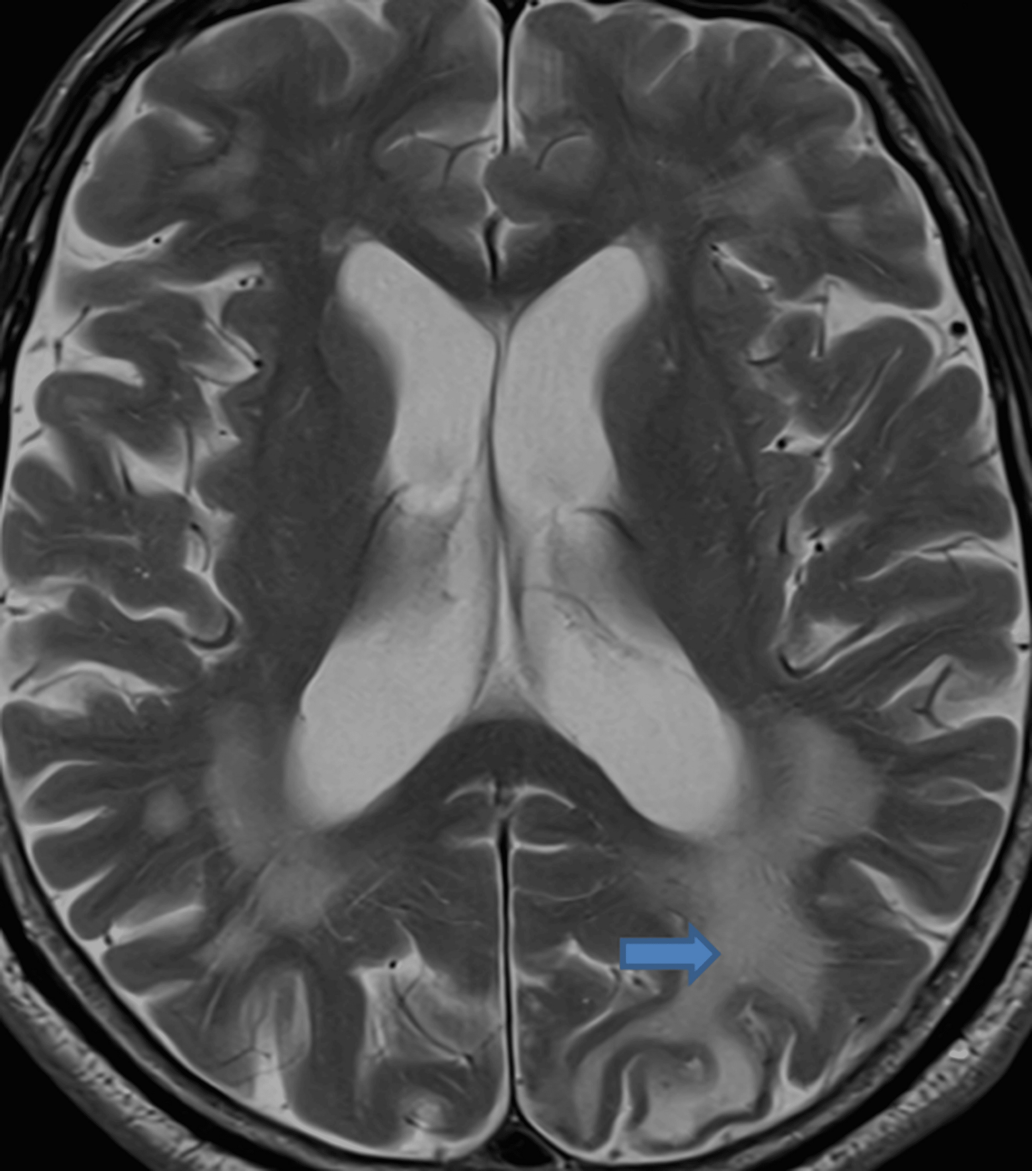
MRI head after six months The arrow is pointing to a marked reduction of hyperintensity in the occipital lobe.
